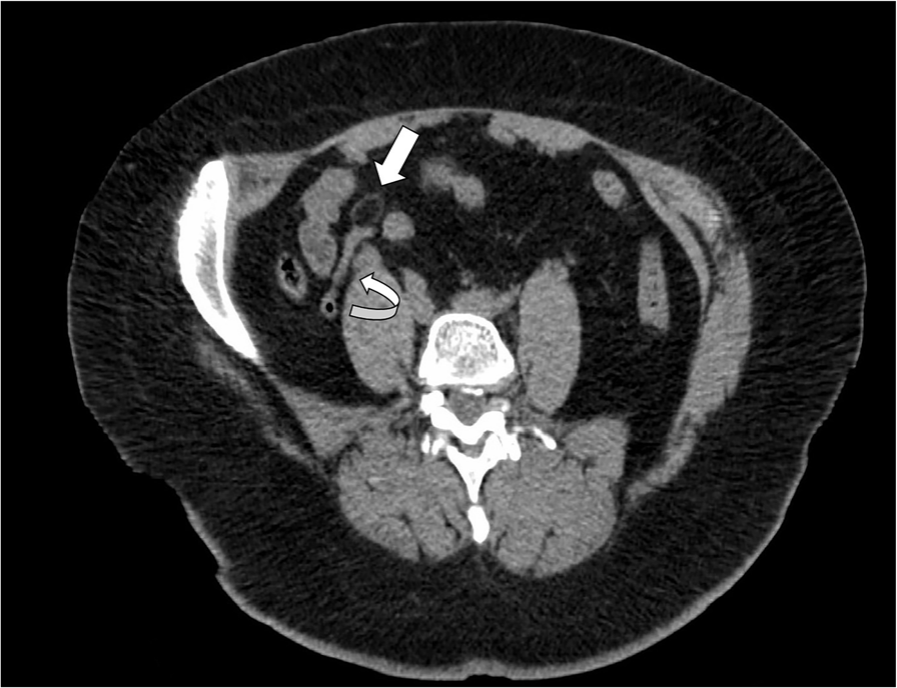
Acute epiploic appendagitis adjacent to the vermiform appendix in a 39-year-old woman. Curved reformatted non-contrast CT image shows an oval lesion with fat attenuation and peripheral hyperdense rim (arrow), that is depending directly from the vermiform appendix tip (curved arrow)
